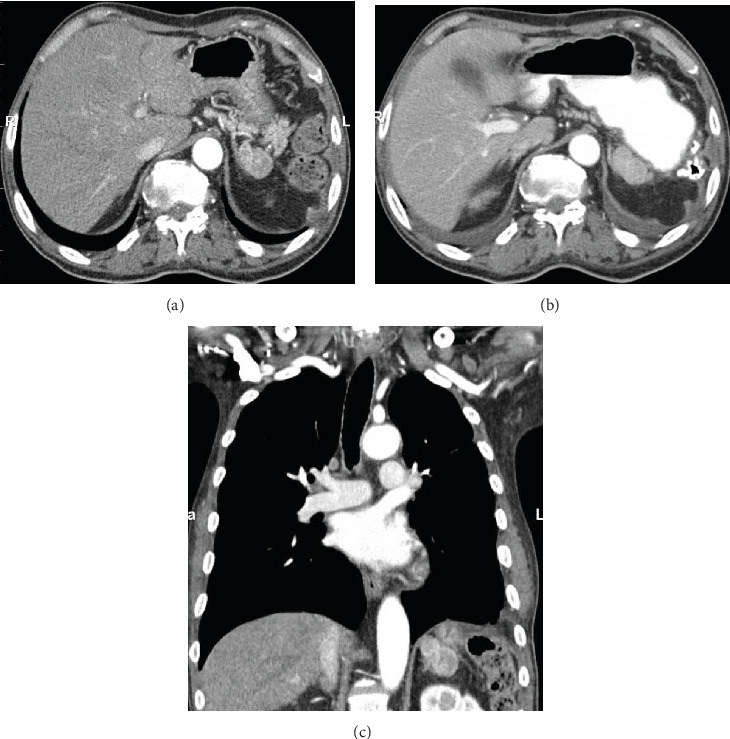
CT scan ((a, b) transverse; (c) coronal view): 5 cm mass close to the tail of the pancreas and next to the left adrenal gland (arrow).
